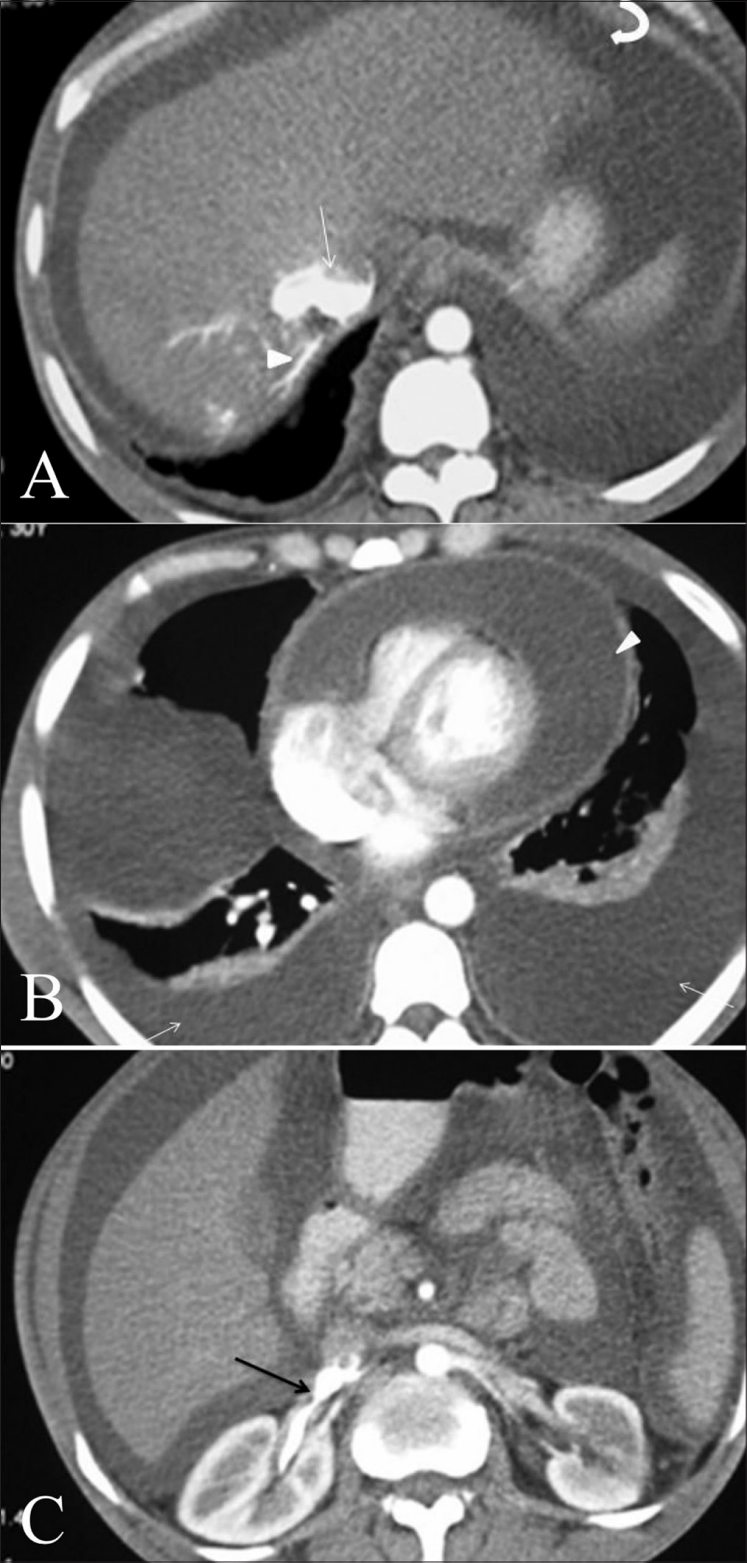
Contrast layering in the abdominal IVC (arrow) and hepatic veins (arrowhead) with ascites (curve arrow) is seen on a contrast-enhanced, axial CT scan (A), in a patient with cardiac tamponade. Gross pericardial (arrow) and bilateral pleural (arrowheads) effusions are seen (B). Contrast layering in also noted in the right renal vein (arrow in C).
